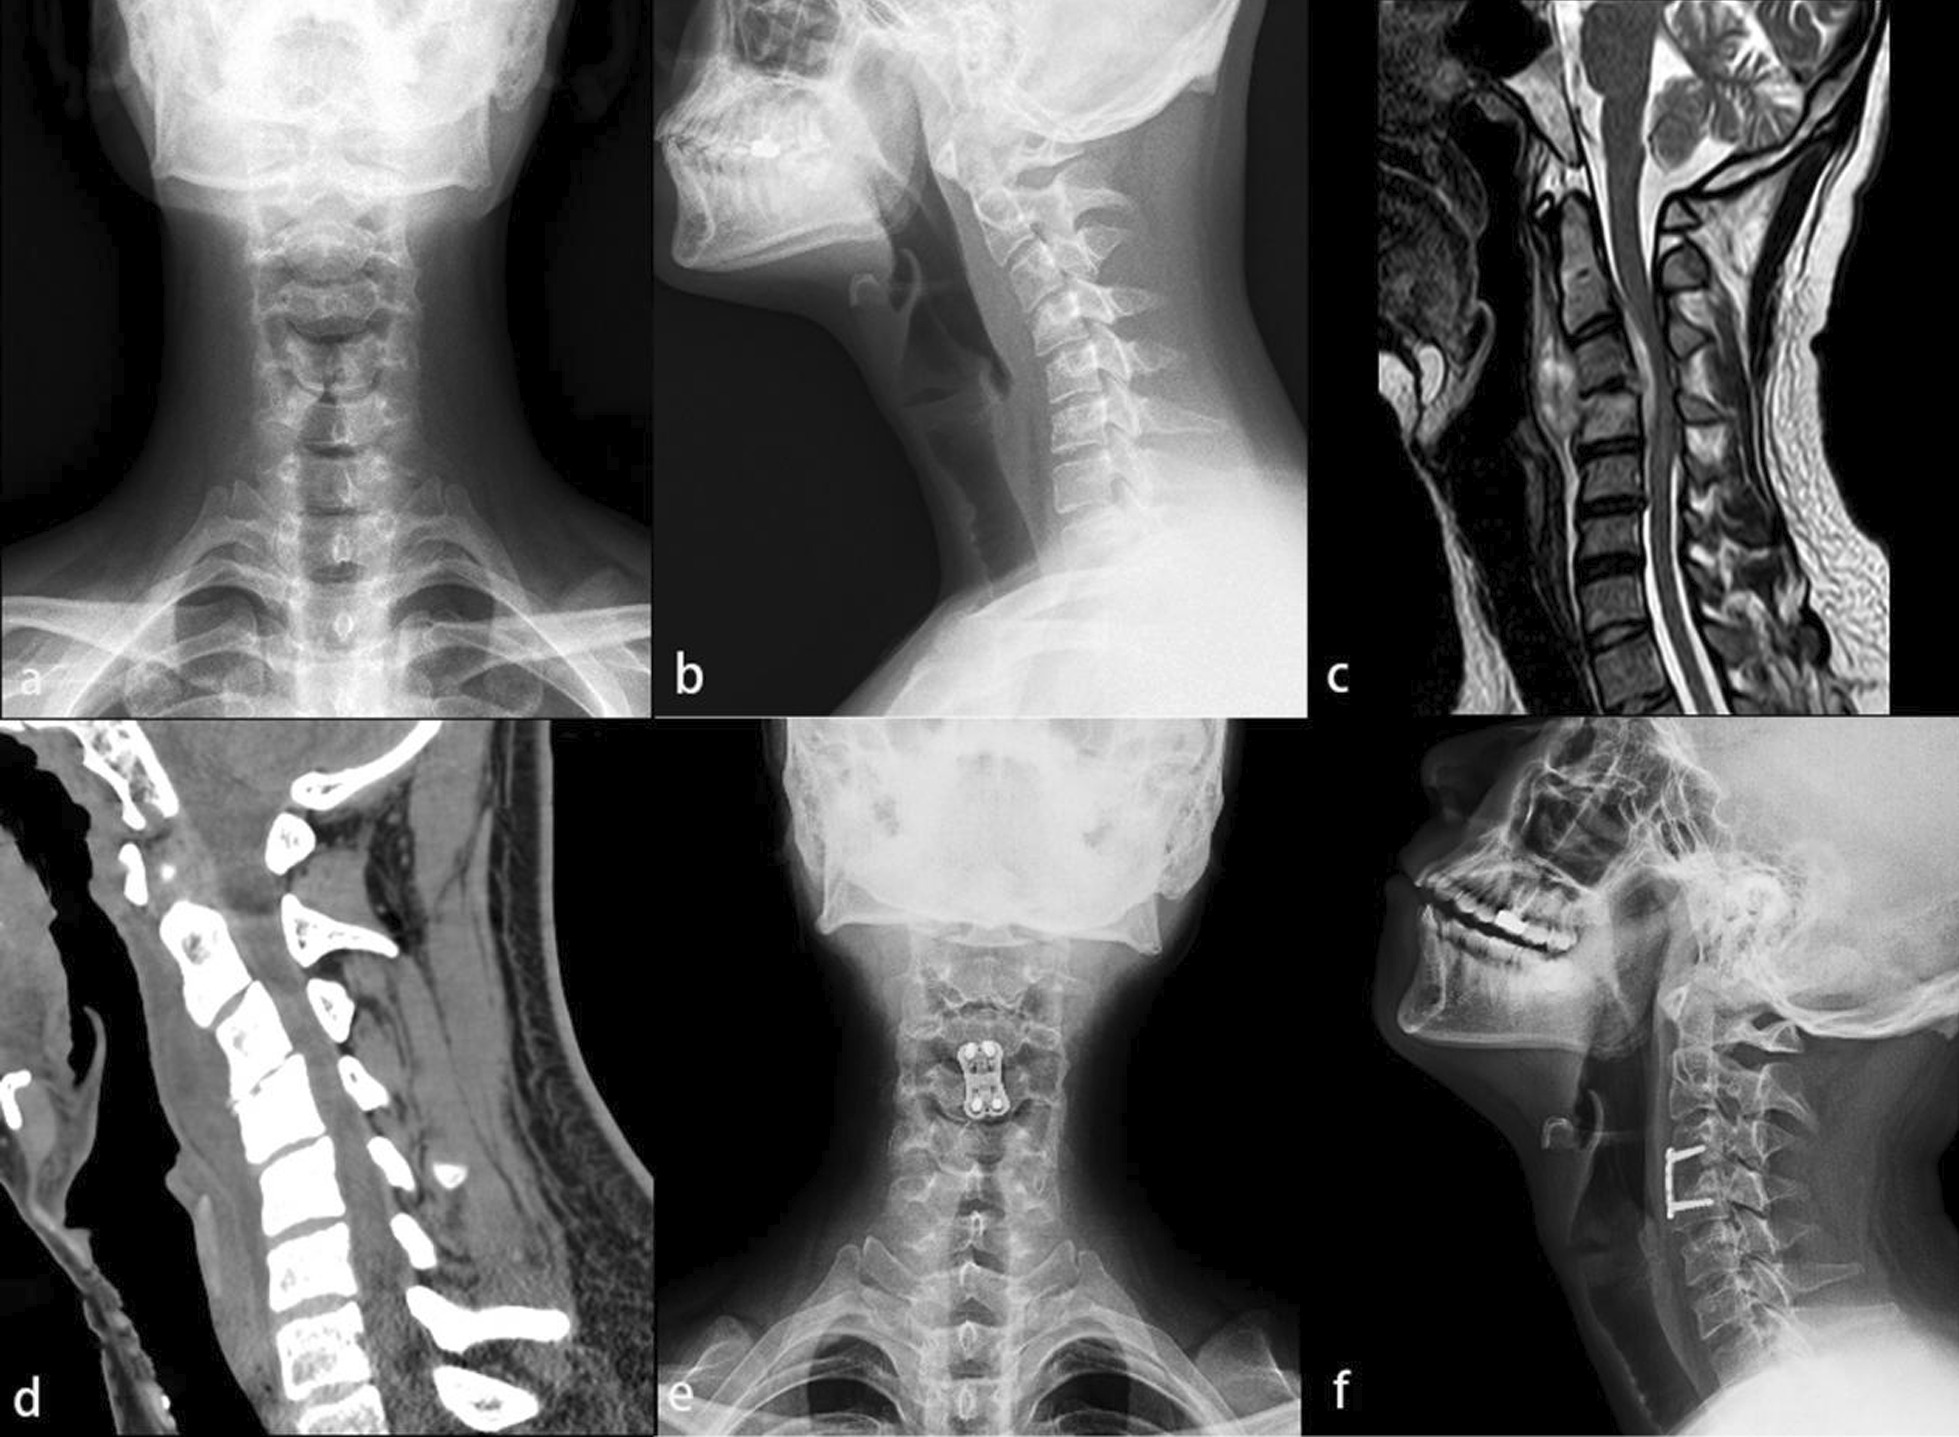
A 27-year-old woman with limited neck pain and mobility for 7 months and progressive limb weakness for 2 weeks. a, b Cervical anteroposterior and lateral X-ray showed C3, C4 vertebral destruction, C2–C4 local kyphosis. c Cervical MRI showed C3, C4 vertebral body partial loss, local kyphosis, and there was a significant abscess in front of the vertebral body. d Cervical CT showed C3 and C4 vertebral destruction. e, f Postoperative anteroposterior and lateral X-ray showed C3 and partial C4 vertebrectomy, the cervical sequence was corrected, and partial bone grafting fusion with stable internal fixation position
